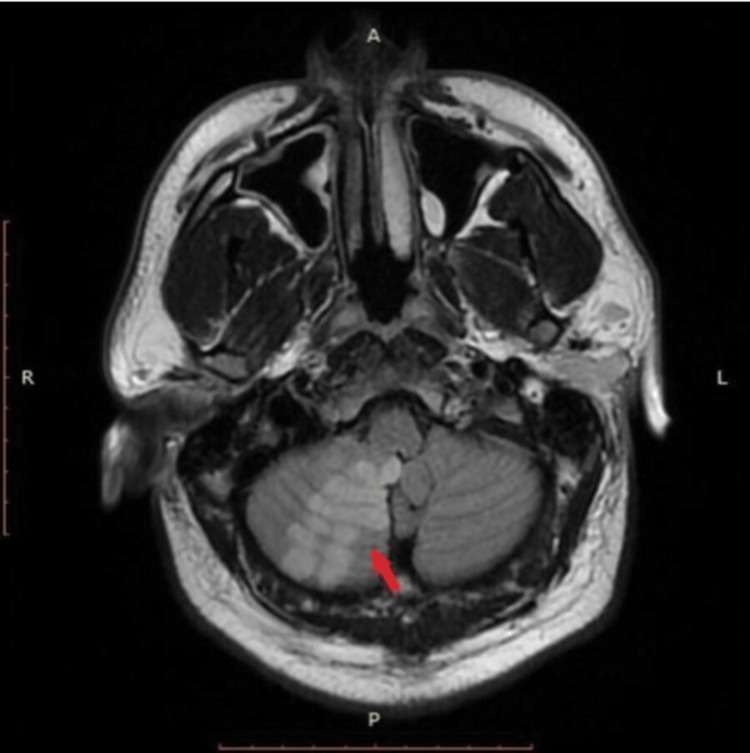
Area of ischemia noted roughly 36 hours after initial presentation in the cerebellum (red arrow) on T2-FLAIR MRI imaging (axial view). The affected area demonstrating higher intensity signal owing to increased amount of fluid from reactive edema. FLAIR: fluid-attenuated inversion recovery
